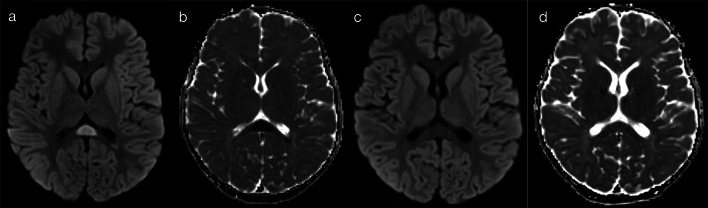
Restricted diffusion in corpus callosum (Pattern 3). 3-year-old child with gastrointestinal illness, followed by lethargy, irritability and suspected focal seizures. Stool was positive for norovirus. DWI (a) and ADC map (b) demonstrated a cytotoxic lesion midline within the splenium. Follow-up MRI 2 weeks later demonstrated complete resolution of the splenial lesion on DWI (c) and ADC (d) images
